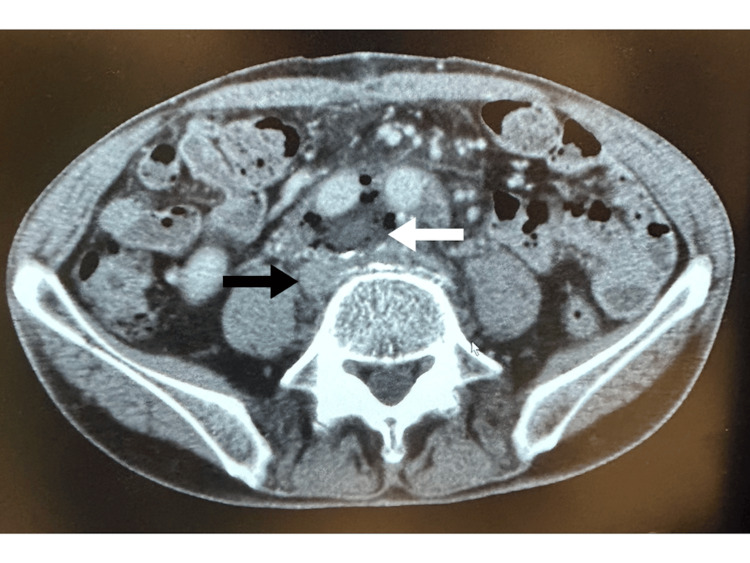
Post-operative computed tomography Post-operative computed tomography scan shows no bleeding or hematoma around the repaired right common iliac vein (black arrow). The white arrow points to the applied Hydrofit^Ⓡ^, arterial patch, and Surgicel^Ⓡ^.
